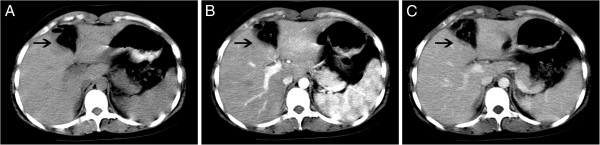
Postoperative computed tomography (CT) findings. In each panel, the arrow indicates the operative site. (A) Unenhanced phase CT image. (B) Arterial-dominant phase CT image. (C) Portal-dominant phase CT image.
